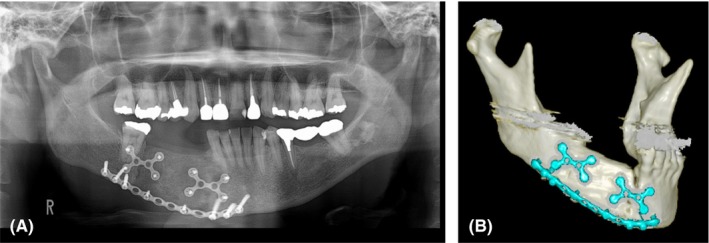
A, Postoperative orthopanoramic radiograph. B, The right mandible continuity defect was reconstructed with nonvascularized free iliac bone to correspond to the computed tomographic image
